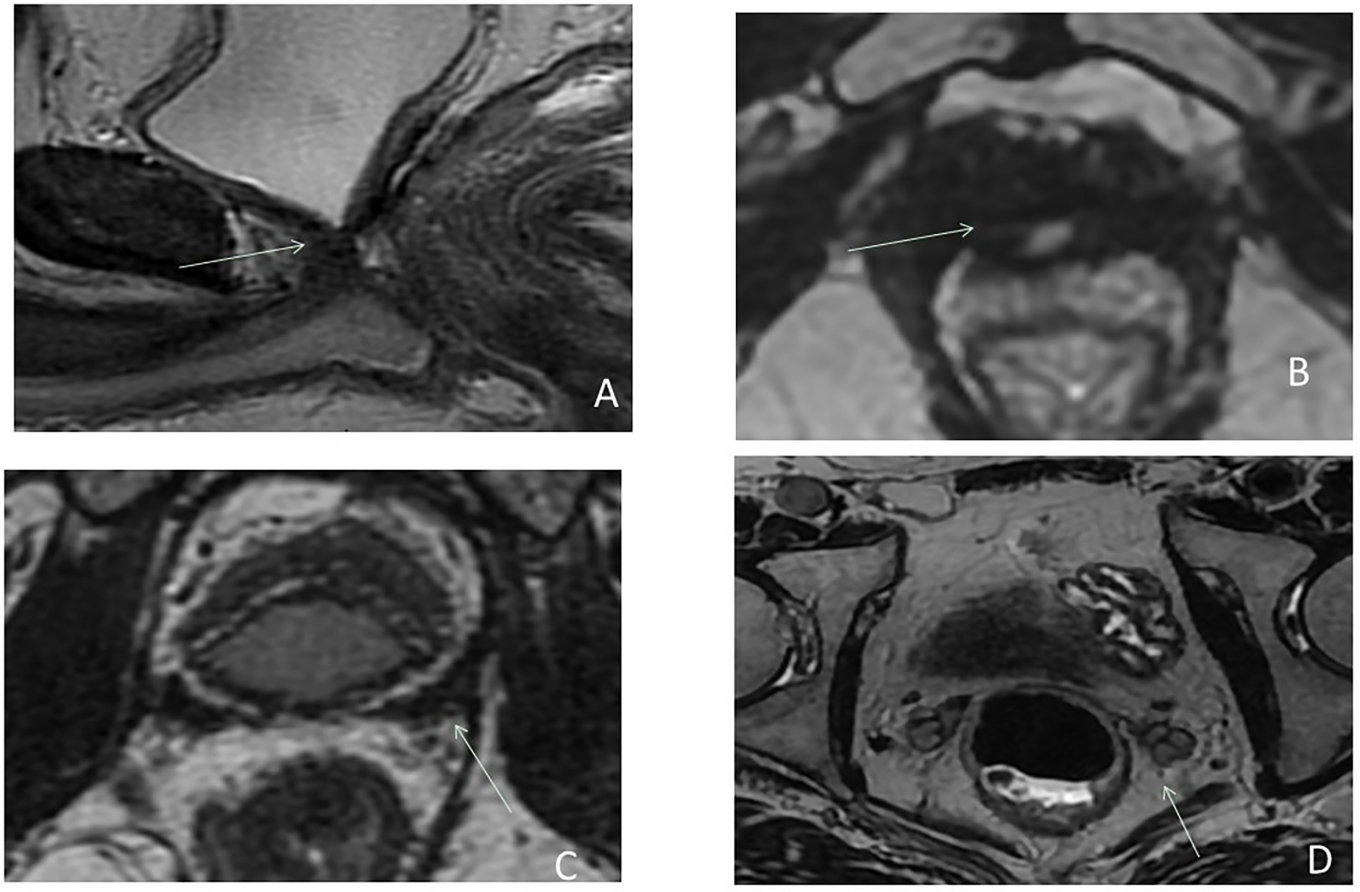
Postsurgical imaging findings after radical prostatectomy on sagittal (A) and axial (B–D) T2-weighted imaging: bladder neck descended into the prostatectomy fossa with a more conical shape (A); the VUA anastomosis (arrow) (A, B) visualized as a ring of postoperative fibrosis on low signal intensity inferior to the bladder neck. Seminal vesicles which are supposed to be removed in a classical RP demonstrate a low signal intensity (C) (postoperative fibrosis), but may be retained in part and can be seen in their presurgical locations with their characteristic tubular structure on T2W (D).
